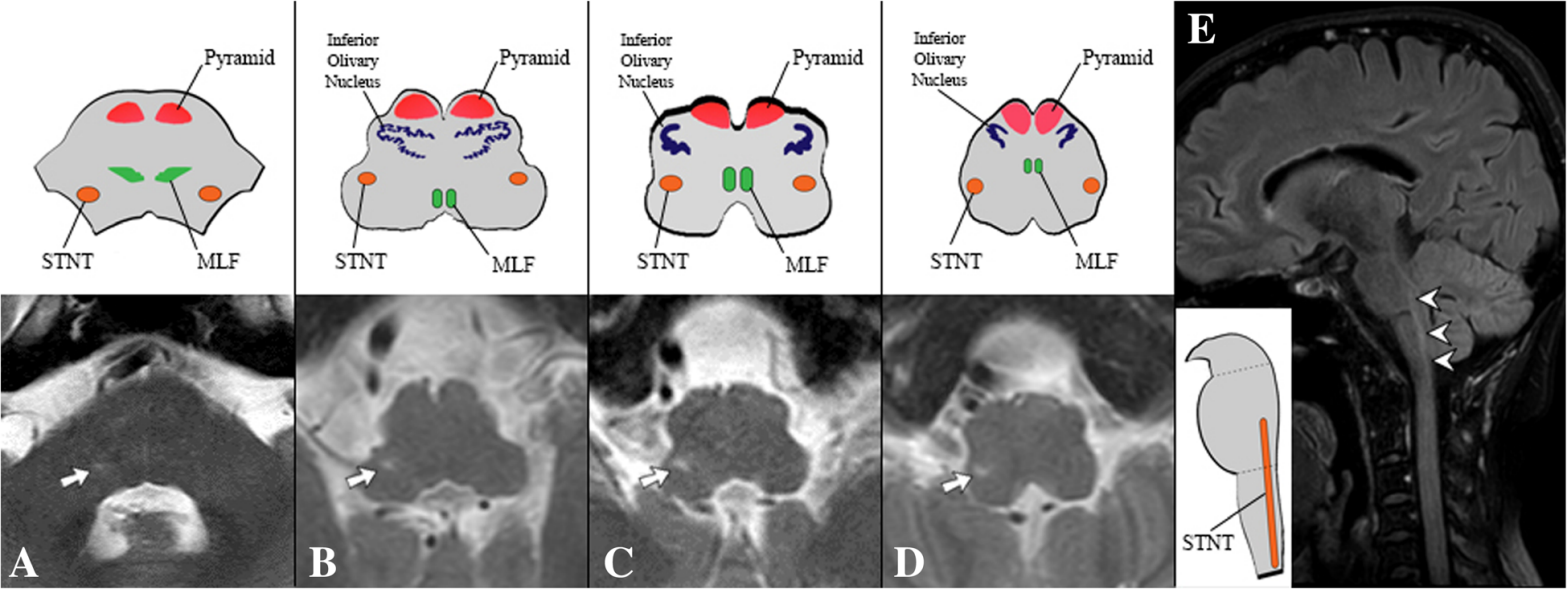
Magnetic resonance imaging of the brain*.* Axial T2 weighted shows hyperintense T2 lesions (arrows) extending from right dorsolateral pons to the medulla (a-d). Sagittal fluid attenuation inversion recovery (FLAIR) shows linear, vertical-oriented hyperintense lesions (arrowheads) (e). These lesions represent the anatomical location of the spinal trigeminal nucleus and tract (STNT) along the brainstem. MLF, medial longitudinal fasciculus
